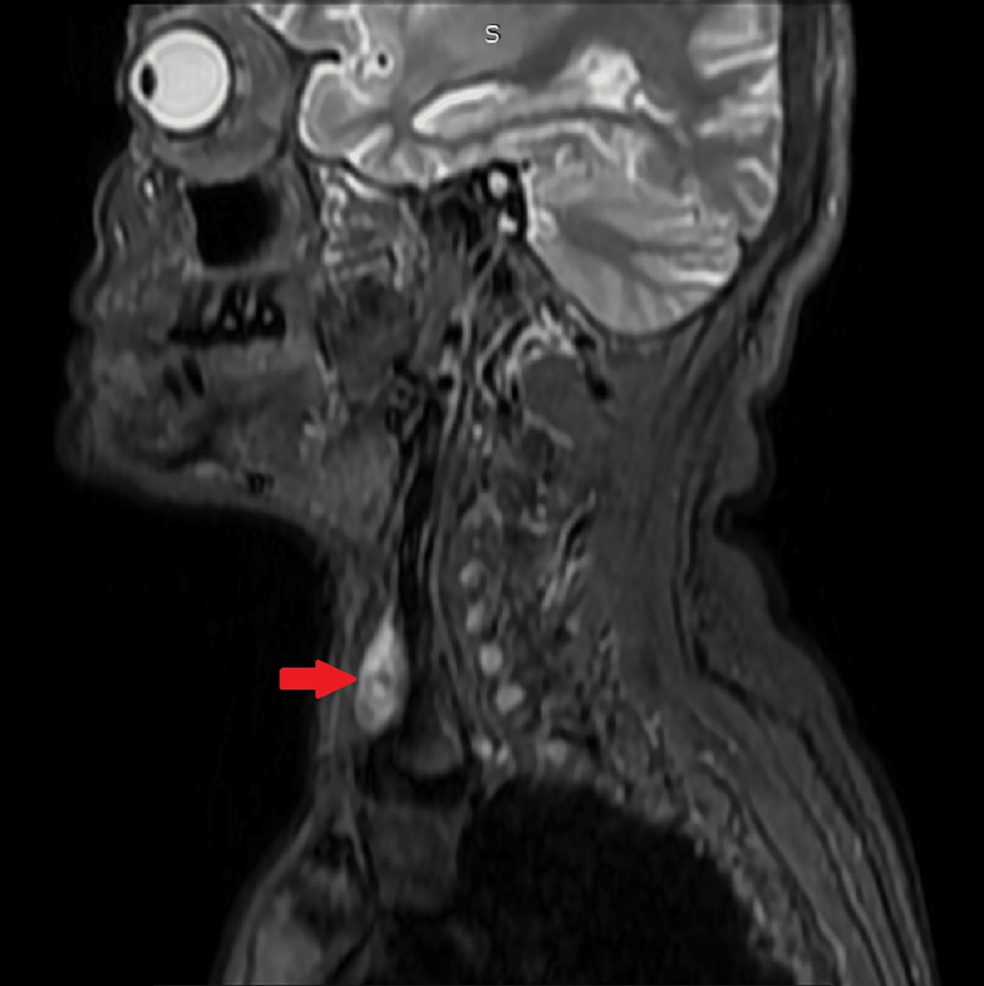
MRI of the neck in sagittal view (STIR) A focal, well-defined, heterogeneously hyperintense lesion can be seen (red arrow), consistent with parathyroid adenoma. STIR: short inversion time inversion recovery
